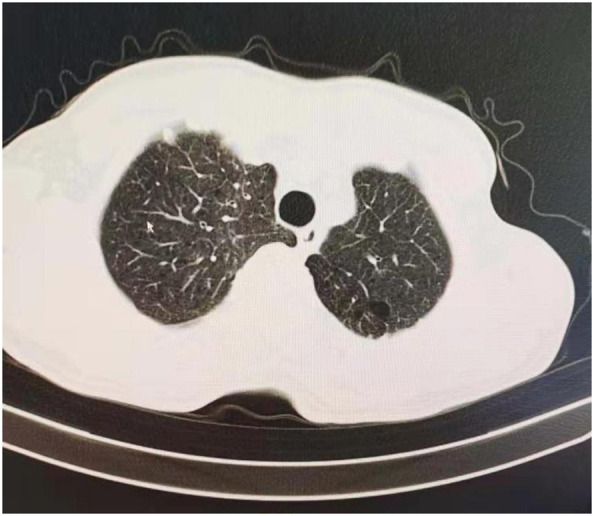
CT screening of included patient, this case had pulmonary bullae located at left upper lobe.
